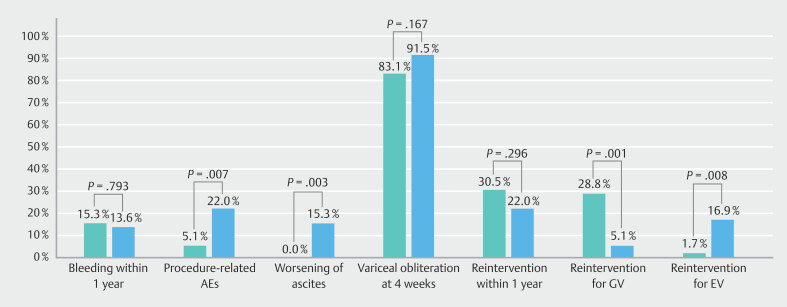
Clustered columns comparing primary and secondary outcomes between endoscopic ultrasound (EUS) and retrograde transvenous obliteration (RTO). Highlighted values indicate statistically significant differences.
